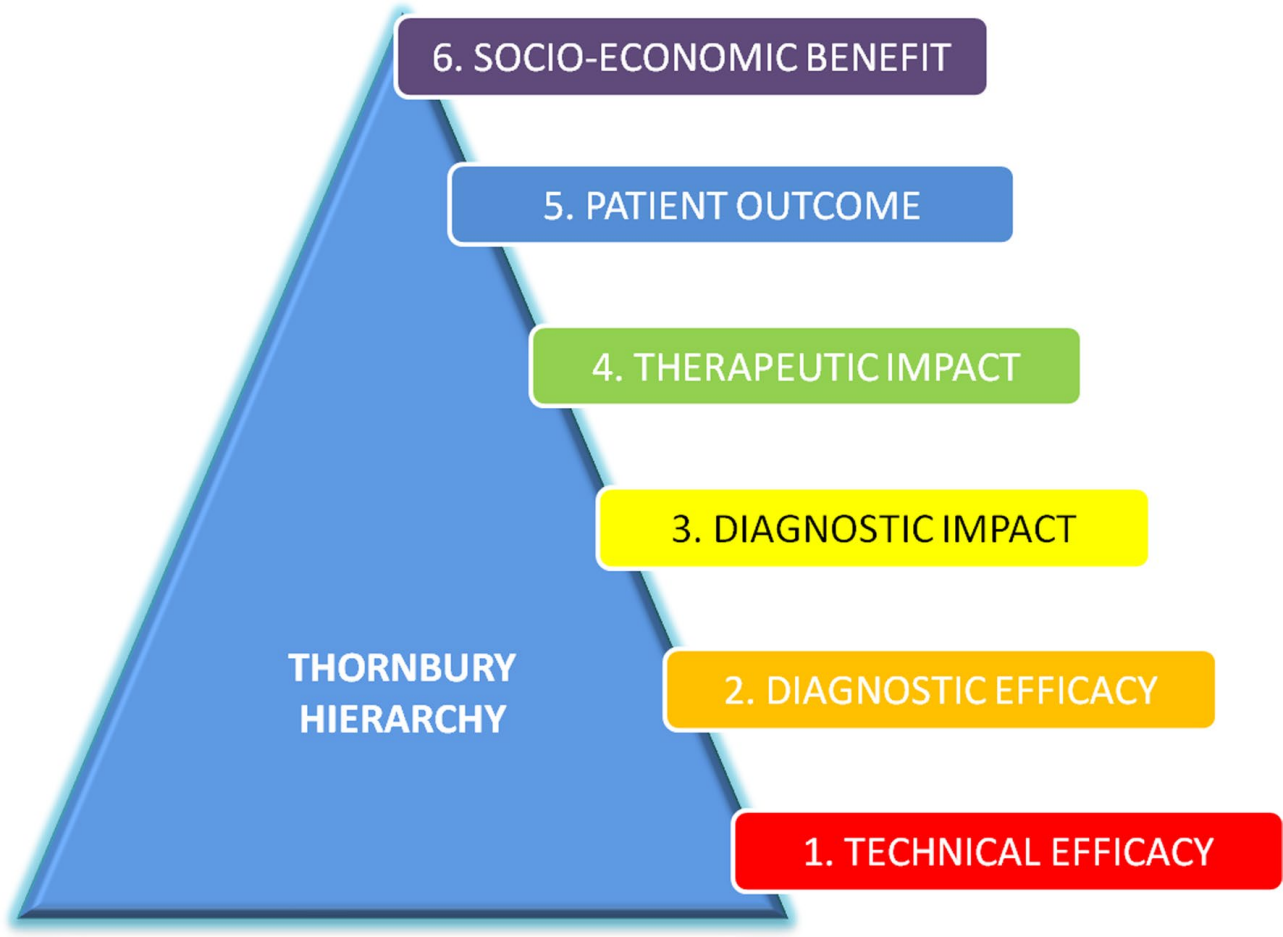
Hierarchical value model. (Reproduced with permission from Raja UA, Patel S, Singh LK, Shah D, Hamdulay S, Penn H, Remedios D. Early arthritis ultrasound: a 4-year outcome study. ECR 2014, EPOS, 10.1594/ecr2014/C-2059)
